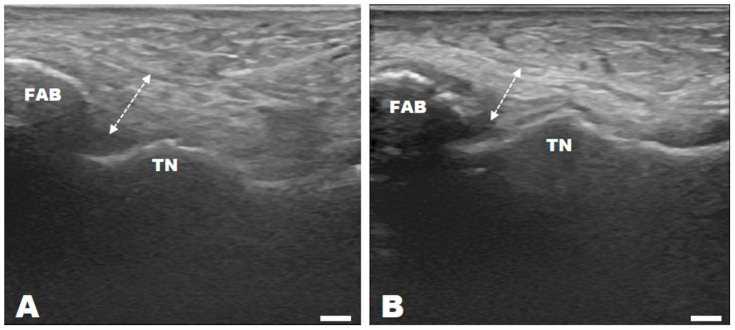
Ultrasonography for the extensor retinaculum and anterior talofibular ligament. The double dashed arrow is a musculoskeletal ultrasound image of the extensor retinaculum and anterior talofibular ligament thickness measurement (scale bar = 5 mm). (A) Ultrasound image after 6 weeks of AMBO, (B) ultrasound image after 12 weeks of AMBO and after balance exercise for 6 weeks. AMBO, arthroscopic modified Broström operation, FAB: fibula anterior border, TN: talus neck.
